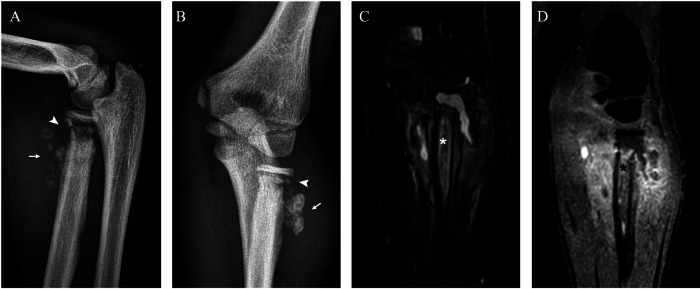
Neuropathic arthropathy of the left elbow of a 9-year-old boy with hereditary sensory and autonomic neuropathy type IV. Lateral (A) and anteroposterior (B) radiographs show lysis of the radial proximal metaphysis (arrowhead) and bone fragments (arrow). Coronal proton density (C) and post-gadolinium MRI (D) show oedema (white asterisk) and enhancement (black asterisk) of the proximal radius and surrounding soft tissues
